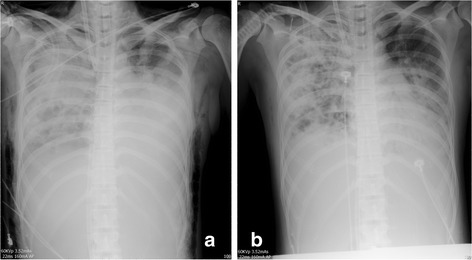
Chest X-ray on day 7 (a) before ECMO, HFOV and prone position combination therapy and on day 9 (b) after the combination therapy
